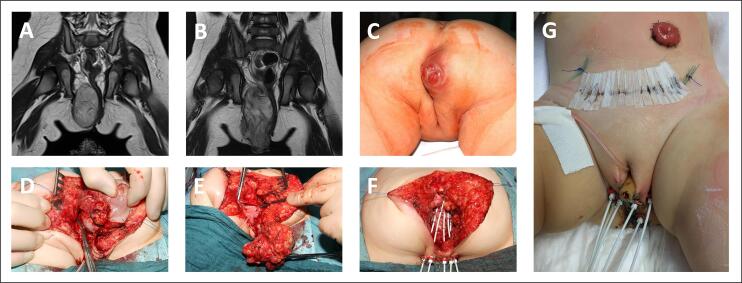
Radical resection combined with brachytherapy in a 14-month-old girl (patient #3) with embryonal, perianal rhabdomyosarcoma. A) Initial MRI. B) Preoperative MRI. C) Tumor localization. D) Situs with tumor and opened dorsal rectal wall (probe in rectum). E) Situs with tumor and opened dorsal vaginal wall. F) Situs after tumor resection and after placement of brachytherapy tubes. G) Position of brachytherapy tubes, urinary catheter and probe in the vagina, ovaries transpositioned to the anterior abdominal wall.
